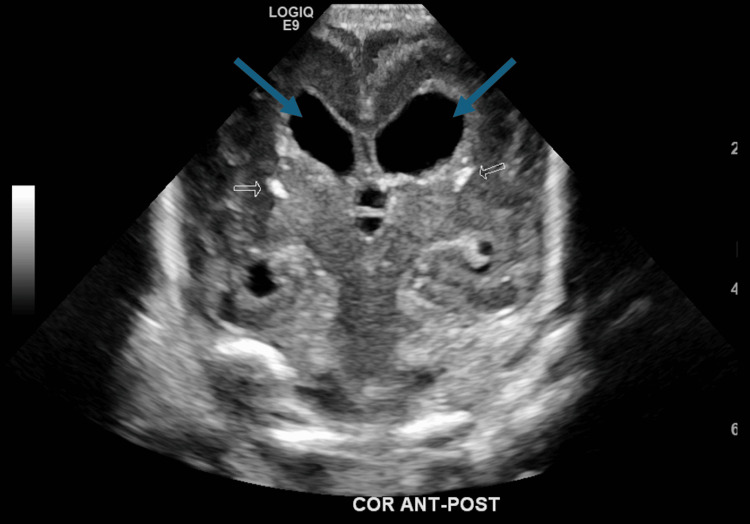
Still image from the head ultrasound on day of life 27 displaying stable ventriculomegaly (blue arrows) and hyperechoic periventricular foci observed bilaterally (white arrows) and prominently seen compared with the previous head ultrasound
